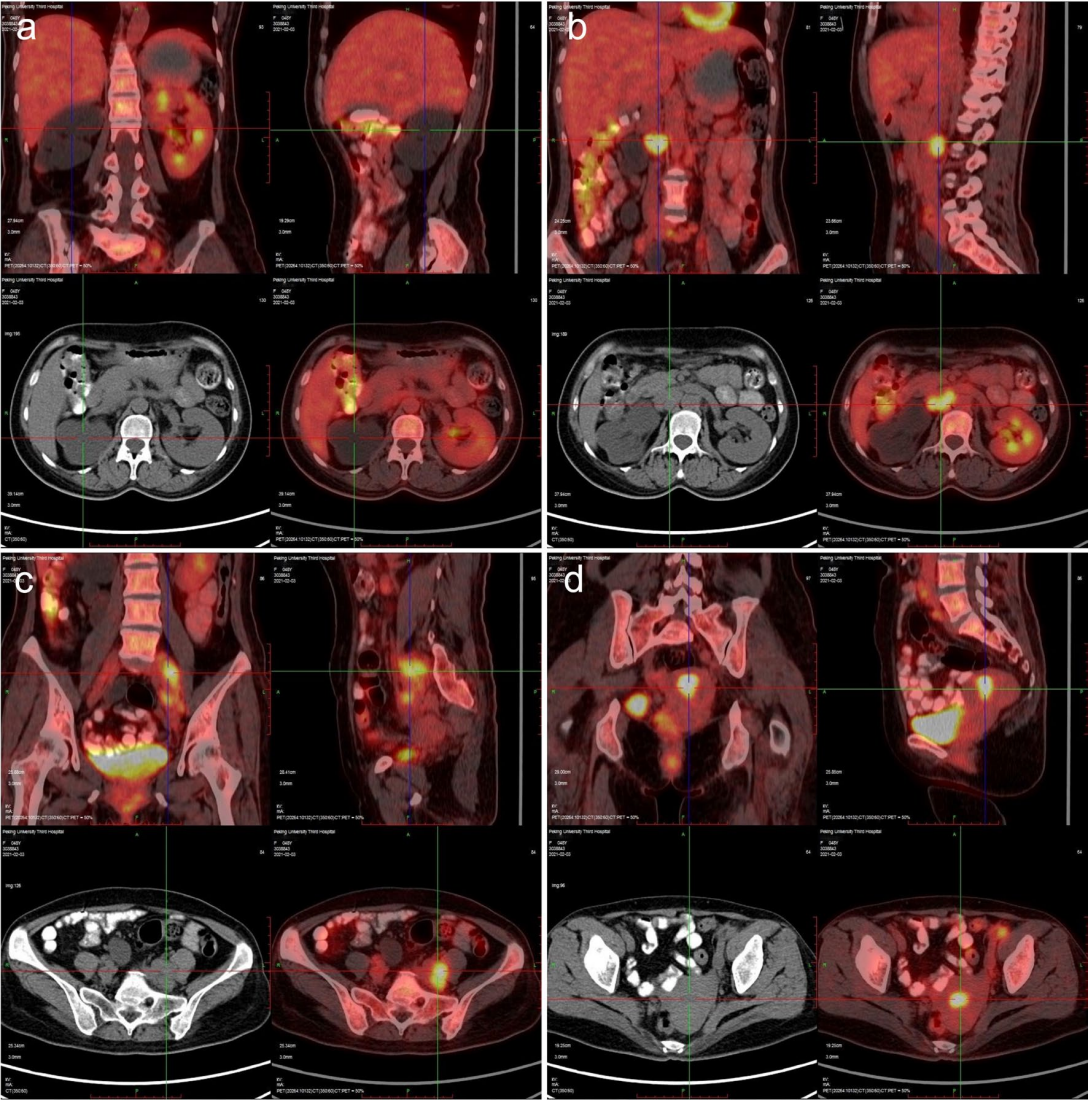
PET/CT findings of various organ involvements in this patient were presented. a Renal involvements. The right renal parenchyma was thinned, associated with dilation and hydronephrosis of the right renal pelvis and ureter. b Multiple enlarged lymph nodes with increased FDG uptake in the retroperitoneum, the largest one was about 3.3 × 2.5 cm. c Multiple enlarged lymph nodes with increased FDG uptake in the bilateral iliac vascular areas, the largest one was located on the left side, about 3.4 × 2.0 cm. d Patchy radioactive focus in the uterine cavity
